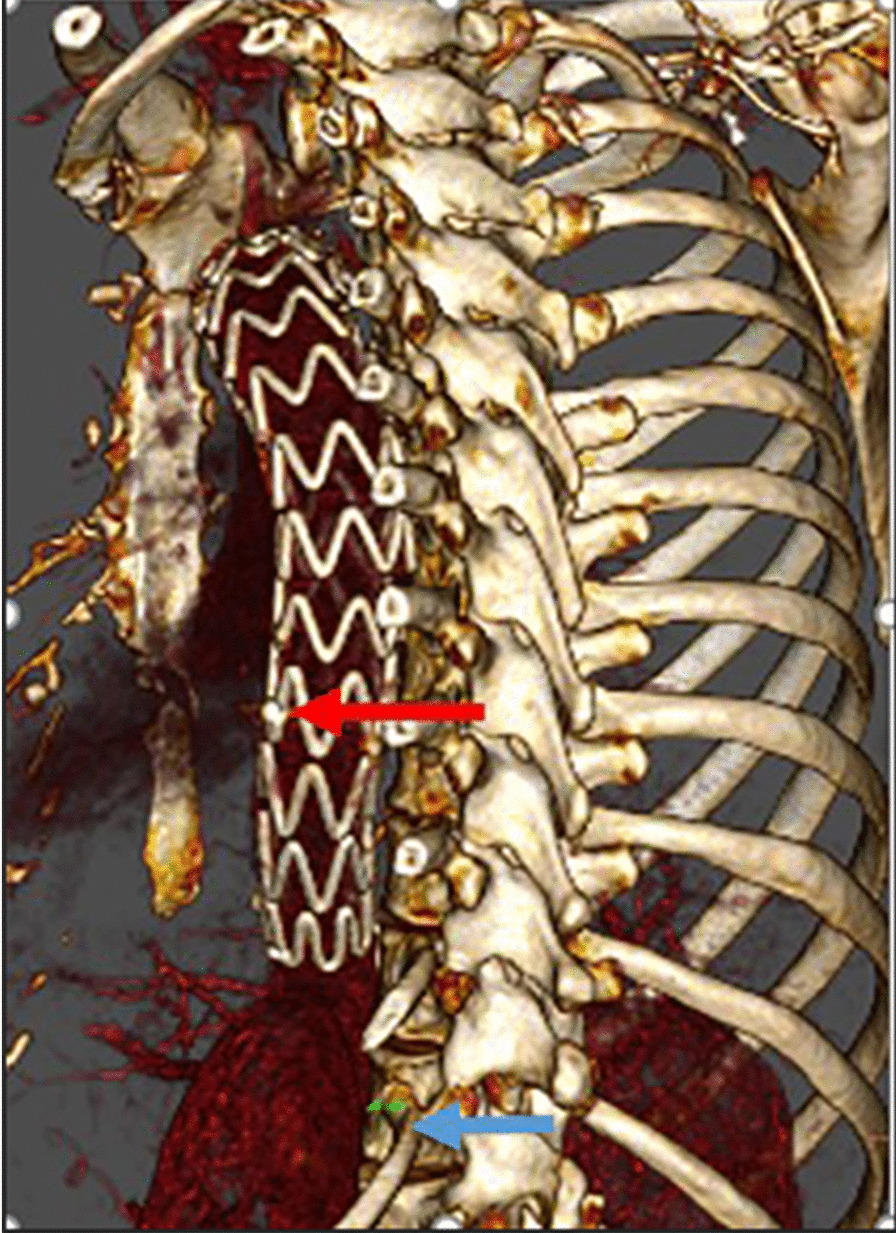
Evaluation of the marker indicating the starting position of the multi-perforated segment and thoracic vertebra. All patients were evaluated through the 3D reconstruction of CTA postoperative 12 months. The red arrow points to marker, and the blue arrow points to T12
